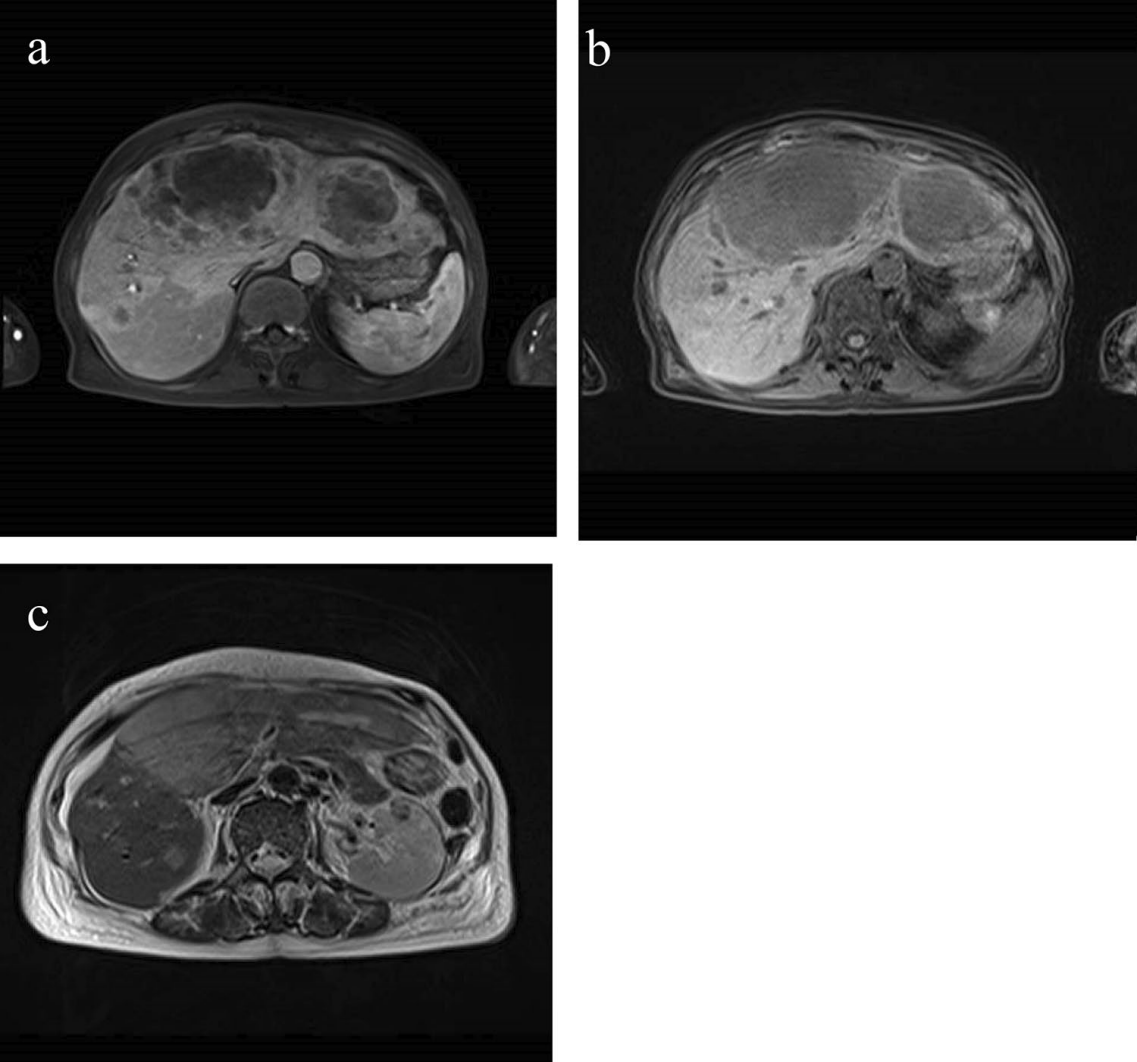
Gadolinium–ethoxybenzyl–diethylenetriamine pentaacetic acid (Gd–EOB–DTPA)-enhanced magnetic resonance imaging of abdominal region. a Liver tumor at arterial phase of enhancement. b Liver tumor at hepatobiliary phase. There was faint contrast around the tumor, and almost contrast effect was not observed in the center of the tumor same as CT scan. c Renal tumor. The tumors with poor contrast enhancement was observed
